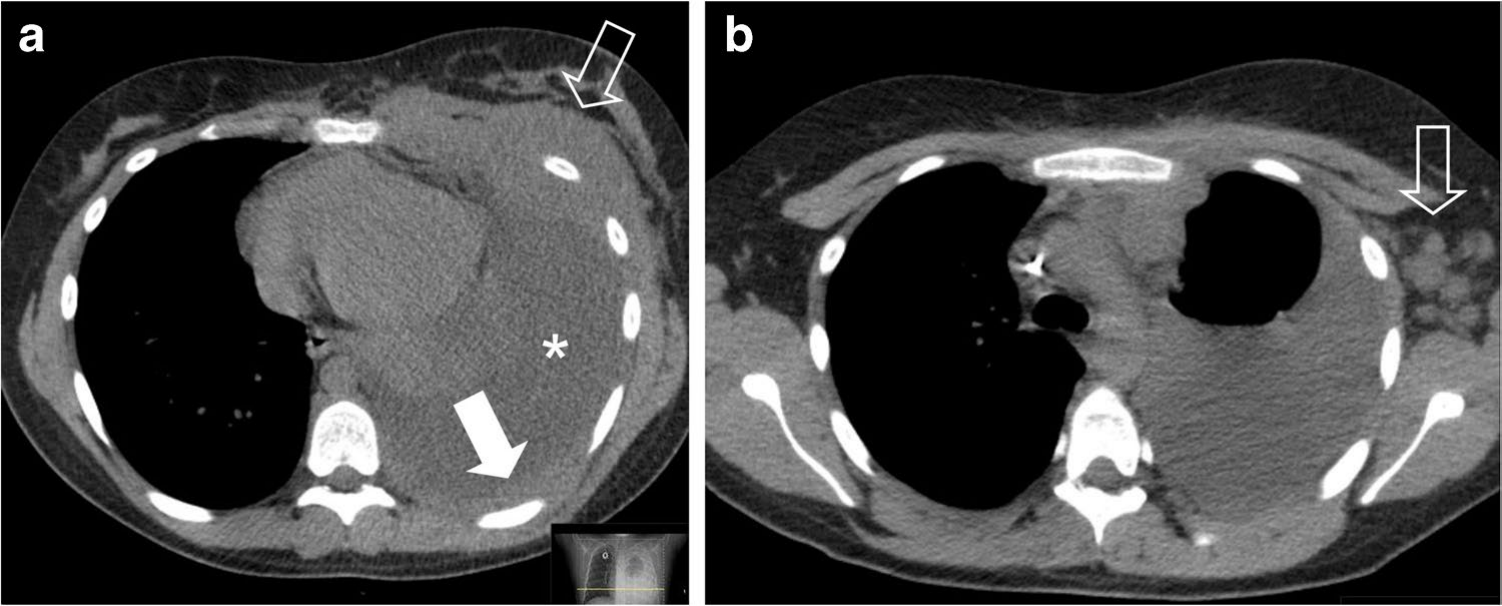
a, b Axial non-contrast enhanced chest computed tomography scans of a 15-year-old girl (patient 2) with rhabdomyosarcoma show (a) a large soft-tissue mass surrounding multiple ribs (*open arrow*) with extension into the chest cavity (*solid arrow*) leading to a malignant pleural effusion (*asterisk*) and (b) multiple axillary lymph node metastases (*arrow*)
